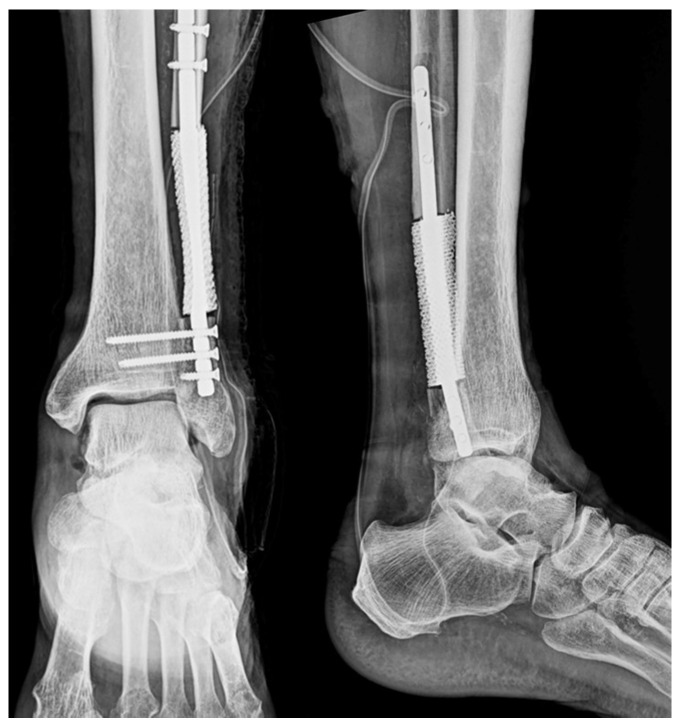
Postoperative imaging. Postoperative radiographs of the ankle in anteroposterior and lateral views showing stable intramedullary prosthesis fixation and proper alignment.
